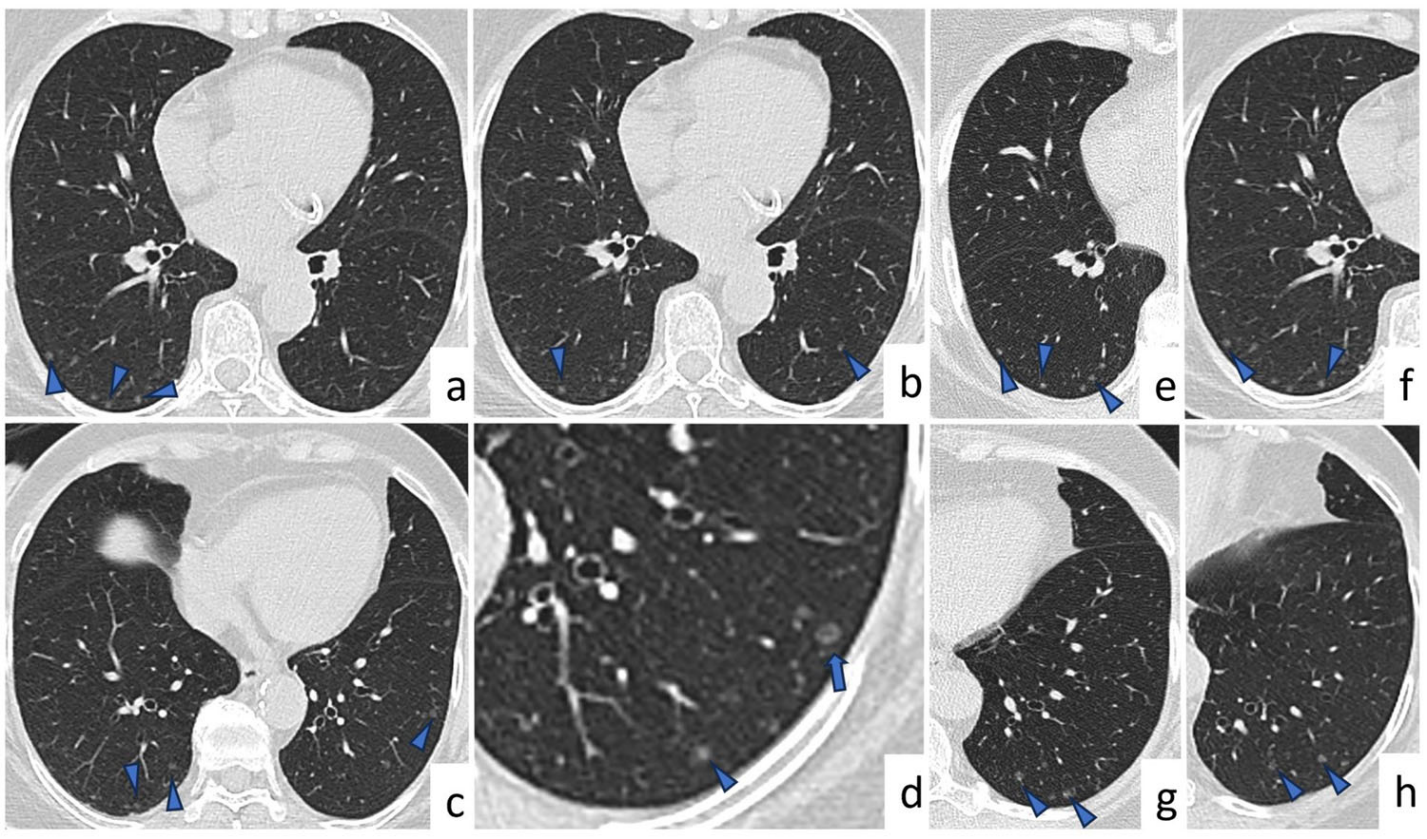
Minute pulmonary meningothelial-like nodules. A 61-year-old female patient suffering from cough and no known history of smoking or malignancy. Axial CT images (a–d) show small, randomly distributed, low-attenuation multiple nodules (arrowheads). Axial CT image of the left lower lobe (d) shows a ring-like nodule with central lucency (arrow) (Cheerio sign). On axial CT images (e–h) in 2007 (e, g) and 2022 (f, h), there is no significant progression in the size and shape of multiple tiny nodules (arrowheads) located in the right lower lobe (e, f) and left lower lobe (g, h)
